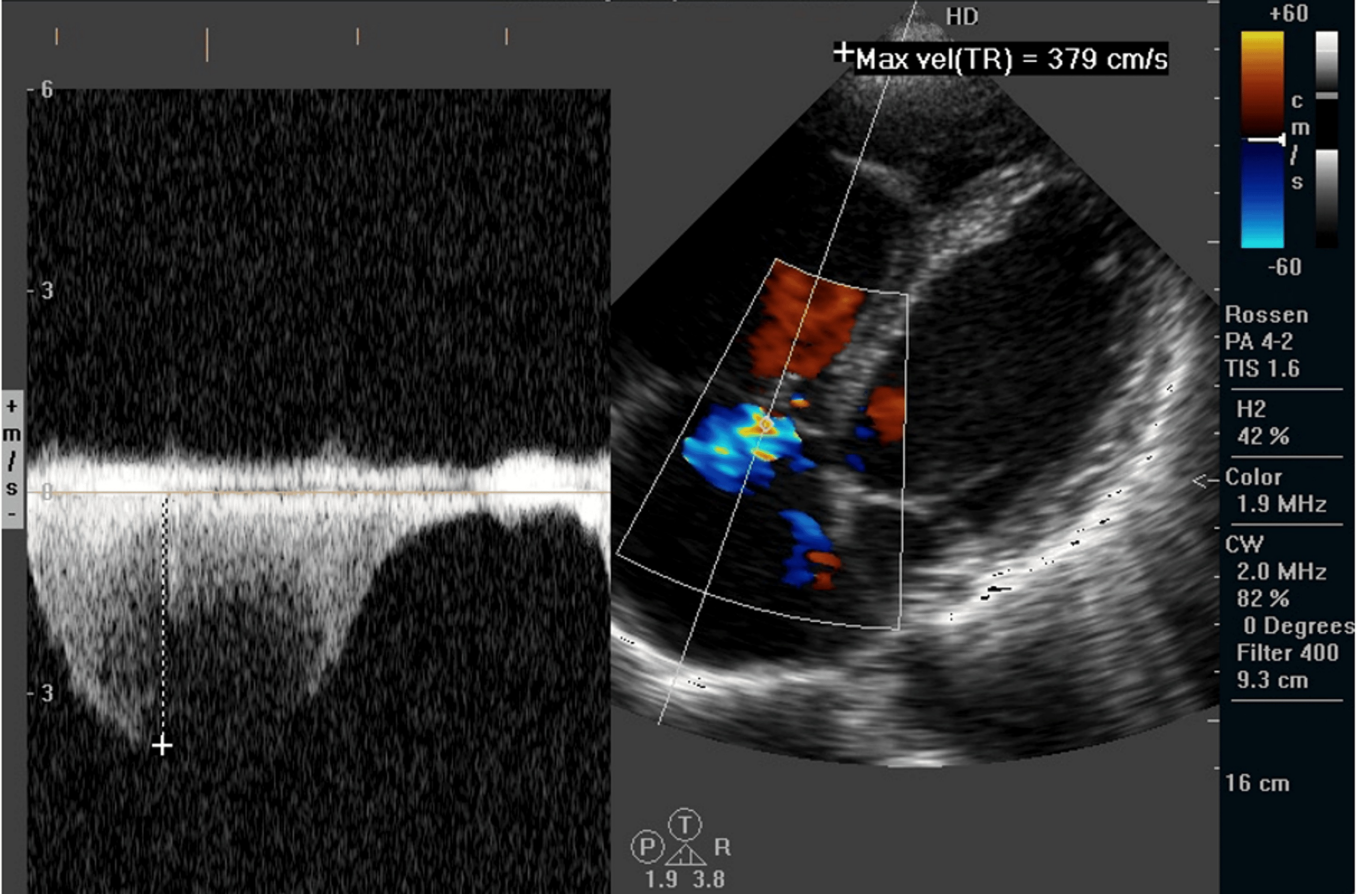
Echocardiography: Tricuspid valve regurgitation, systolic pulmonary artery pressure is calculated at 55 mmHg.
